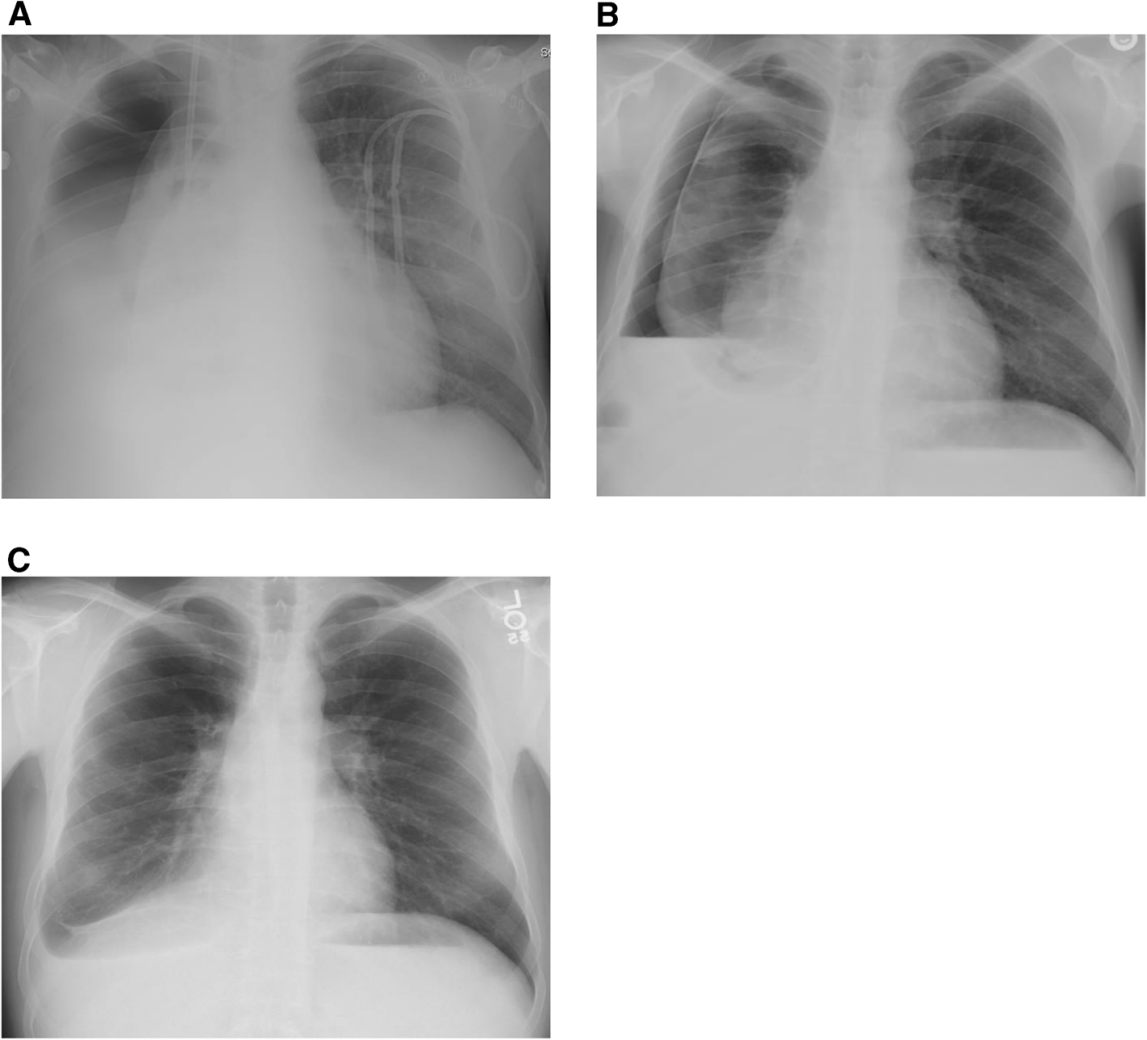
Chest x-ray at discharge with a large right-sided hydropneumothorax (A). Chest x-ray at 1 month after discharge with improvement in the right-sided hydropneumothorax (B). Chest x-ray at 6 months after discharge with significant right lung re-expansion with a small residual hydropneumothorax (C).
